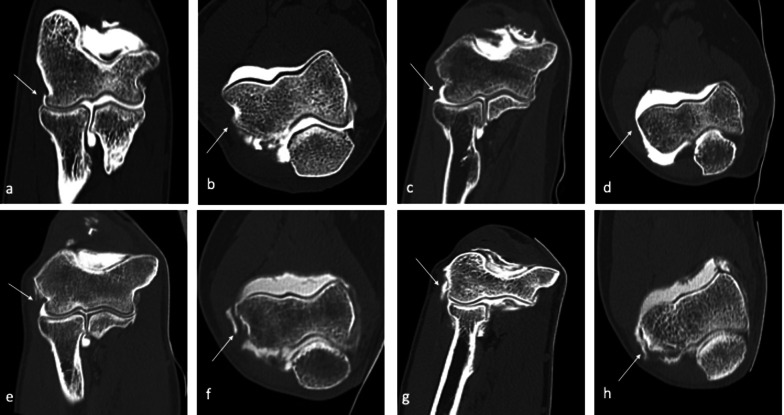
CT-arthrography coronal (a, c, e, g) and axial (b, d, f, h) images belonging to four patients, three of which display different aspects of symptomatic minor instability of the lateral elbow (SMILE), involving the RCL (white arrows). Intact radial band (RCL) of the lateral collateral ligament (LCL) complex (a, b). Widening of the lateral aspect of the articular recess associated to a curve-shaped, loose RCL with no evidence of fiber tearing, suggestive of patholaxity (c, d). Thickened and irregular RCL showing partial-thickness tear (e, f) and full-thickness tear (g, h); iodinated contrast progressively leaks through the ligament, as fibers are gradually disrupted and damage progresses from a partial to a full-thickness tear
